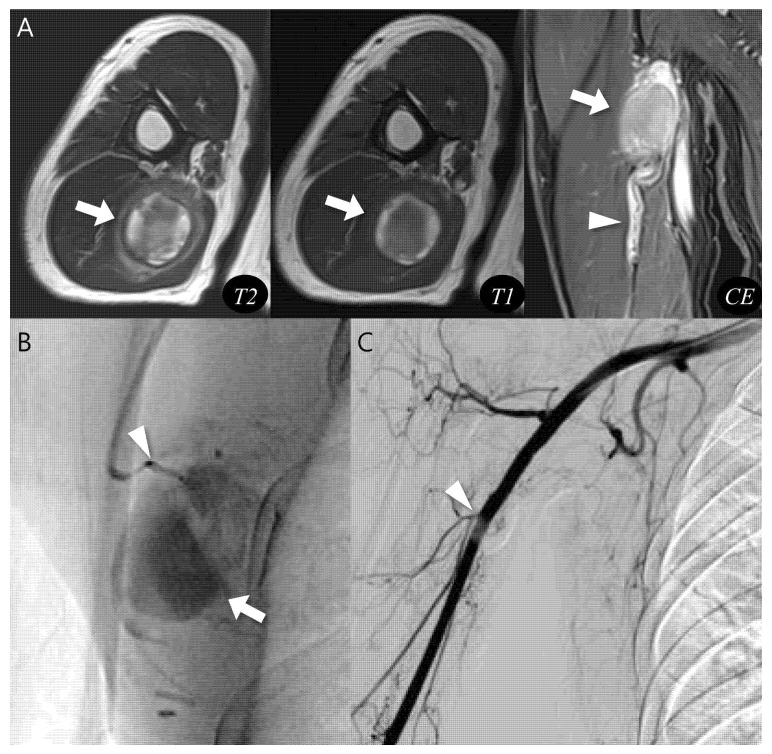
Diagnosis and treatment of pseudoaneurysm. (A) Axial T2-weighted, T1-weighted, and coronal T1-weighted contrast-enhanced MR images show an intramuscular, ovoid lesions (arrow) in the upper arm. The lesion demonstrates a central flow void with inner peripheral high SI on T1-weighted image (arrow, mural thrombus) with neurovascular involvement (arrowhead). (B) Digital subtraction angiography reveals a pseudoaneurysm sac (arrow) with a narrow neck arising from the brachial artery (arrowhead). (C) Post-embolization angiography demonstrates successful occlusion of the pseudoaneurysm neck (arrowhead).
